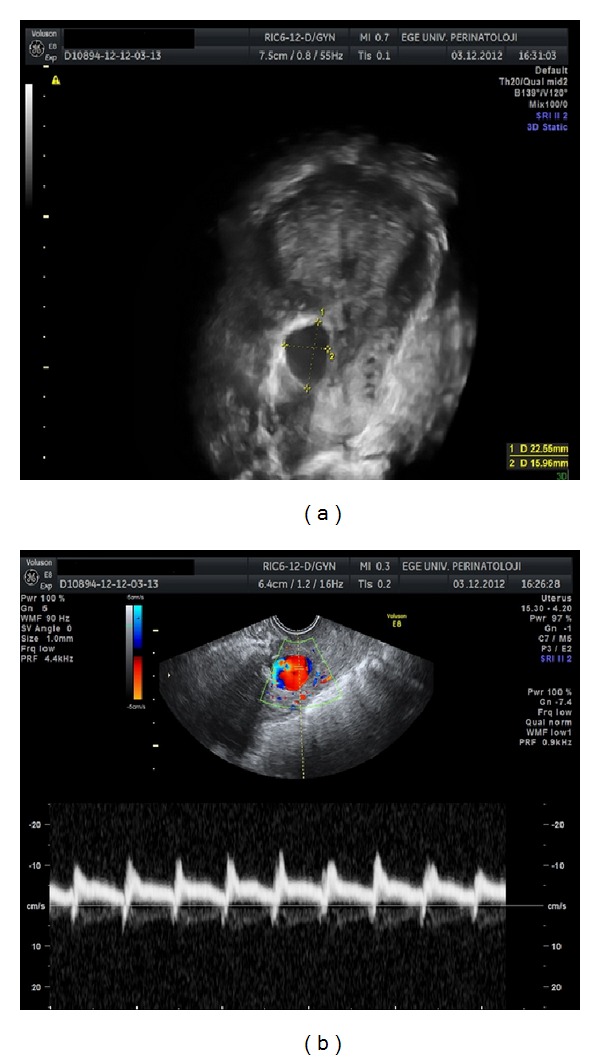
A cystic mass was observed on the right lateral isthmic region with a size of 22 × 16 mm on gray scale and 3D sonography. Color flow and spectral Doppler imaging of the cystic mass revealed marked aliasing and bidirectional flow representing systolic and diastolic blood flow.
